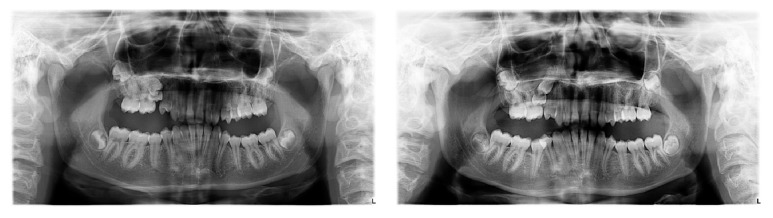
Panoramic radiographs of the identical twins.
